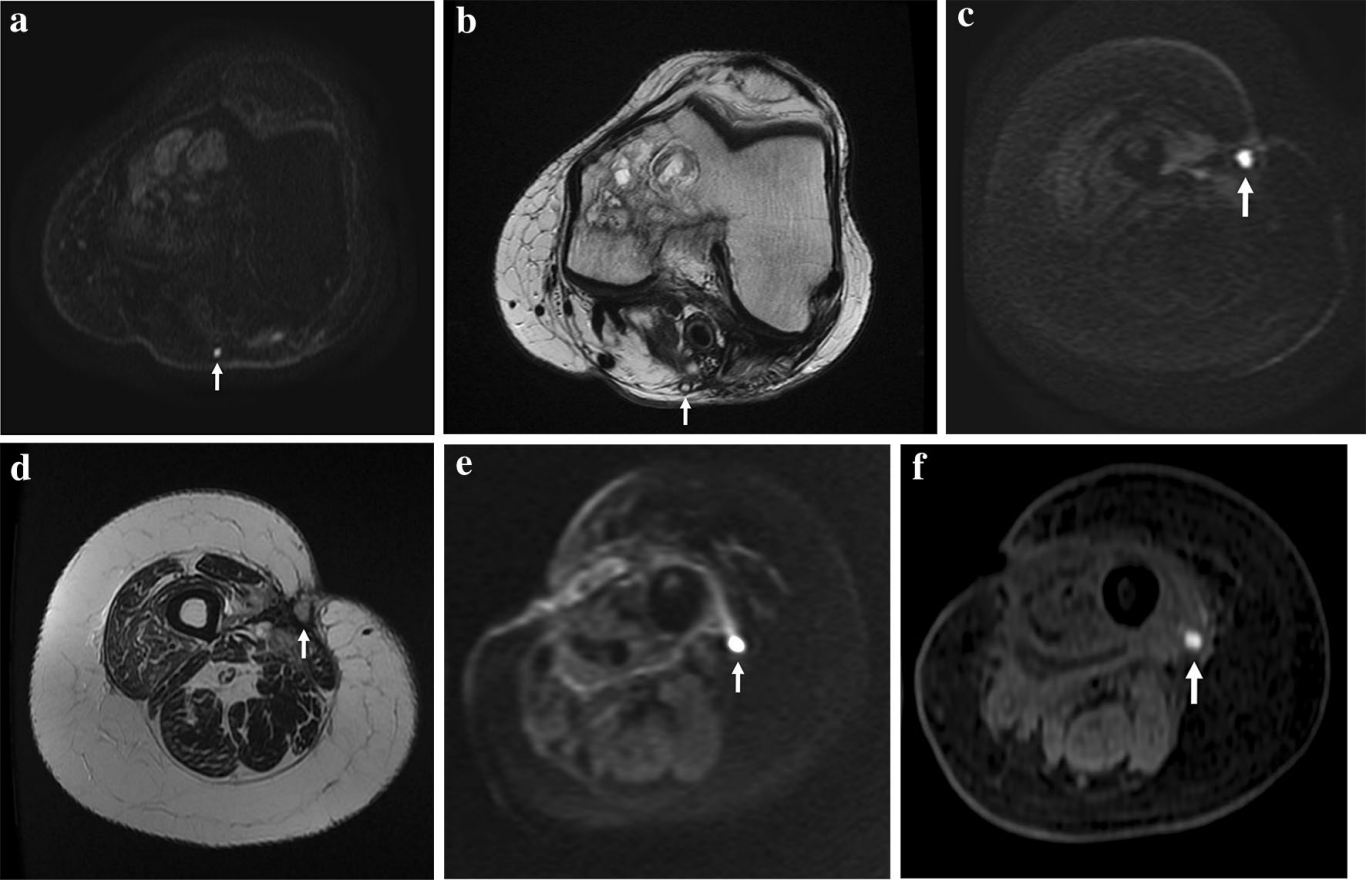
Examples of non-tumoral hyperintense nodules on DWI. Focally dilated vein (a, b); a axial DWI reveals a focal hyperintensity (*arrow*) posteriorly in the right knee with no diffusivity restriction (ADC = 1.31 × 10^–9^ m^2^/s). b axial T2w image shows a venous structure at the same level (*arrow*). Infective collection (c, d); c Axial DWI depicts a focal hyperintensity (*arrow*) at the level of the scar with diffusivity restriction (ADC = 0.7 × 10^–9^ m^2^/s). d Corresponding axial T2w image shows a subcutaneous infective collection (*arrow*) deeply to a cutaneous fistula. Intramuscular hematoma (e, f). e Axial DWI of the left thigh shows a focal hyperintensity (*arrow*) with diffusivity restriction (ADC = 0.44 × 10^–9^ m^2^/s). f Axial fat-sat GRE T1w shows a focal hyperintensity at the same level of DWI finding due to methaemoglobin (*arrow*)
